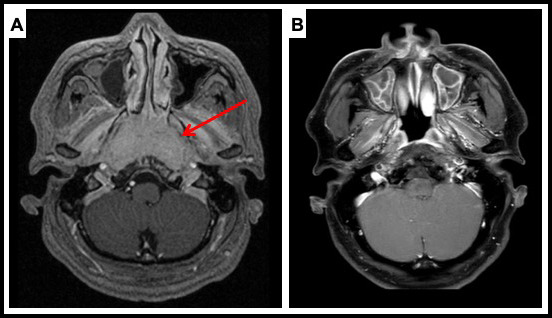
Patient case: 47-year-old male with locally advanced, undifferentiated, nonkeratinizing nasopharyngeal carcinoma (NPC), cT4N2M0 Epstein-Barr virus (EBV)-positive tumor treated with chemoradiation with intensity-modulated proton therapy (IMPT). The IMPT plan is shown in Figure 1. (A) Pretreatment magnetic resonance imaging (MRI; T1 postcontrast magnetization-prepared rapid acquisition gradient echo [MPRAGE] sequence) showing a large nasopharyngeal mass with central skull base invasion. He also had bilateral retropharyngeal and cervical lymphadenopathy. (B) Posttreatment MRI (T1 postcontrast fat sat [saturation] sequence) showing resolution of his extensive nasopharyngeal primary, with some benign posttreatment sinonasal mucosal changes. He had a complete remission (CR) and remains without evidence of disease now 3 years after treatment.
